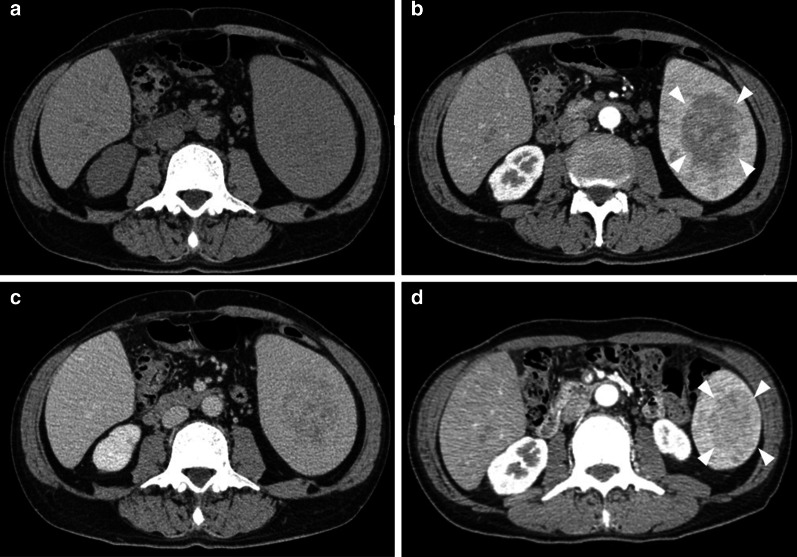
Computed tomography scan of the tumor. A computed tomography scan on admission showed a 70 × 45 mm low-density tumor (a). The tumor exhibited heterogeneous hypo-enhancement during the arterial phase (b arrowheads), and thereafter, the enhancement became gradually homogeneous in the equilibrium phase (c). The tumor was 45 × 30 mm in diameter on a preoperative CT scan 6 months before the surgery (d arrowheads)
